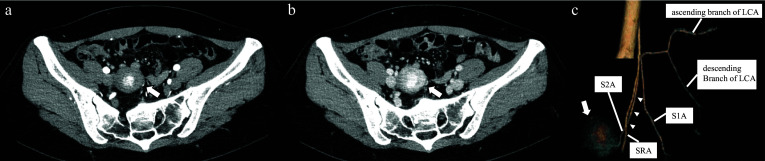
Contrast-enhanced computed tomography (CT) of the abdomen and the pelvis. A 29-year-old woman has a well-defined tumor, 3.5 cm in size, on abdomino-pelvic imaging contrast-enhanced CT. a Central contrast-enhancement in the early phase and b contrast-enhanced margins in the late phase. c 3D-CT angiography showing the mesenteric tumor (arrow) apparently fed by a branch of the S2A (arrow head). *LCA* left colic artery, *S1A* first sigmoidal artery, *S2A* second sigmoidal artery, *IMA* inferior mesenteric artery, *SRA* superior rectal artery
